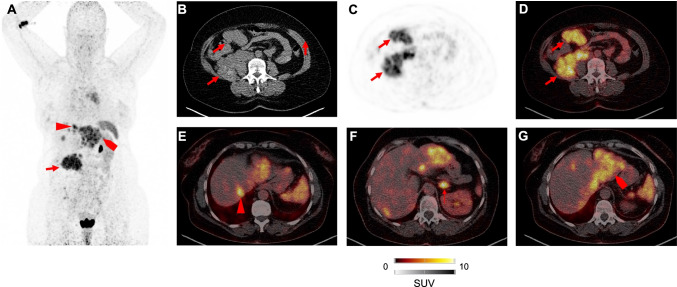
Patient after resection of adrenocortical carcinoma imaged with [^68^ Ga]PentixaFor. The right-sided primary was resected earlier. Maximum intensity projection in A revealed multiple sites of disease after administration of [^68^Ga]PentixaFor. Transaxial CT (B), PET (C), and PET/CT (D) demonstrated intense uptake in a retroperitoneal lesion. Further CXCR4 positive sites of disease included liver lesions (E and G). Additional discernible uptake in the left adrenal gland (F)
